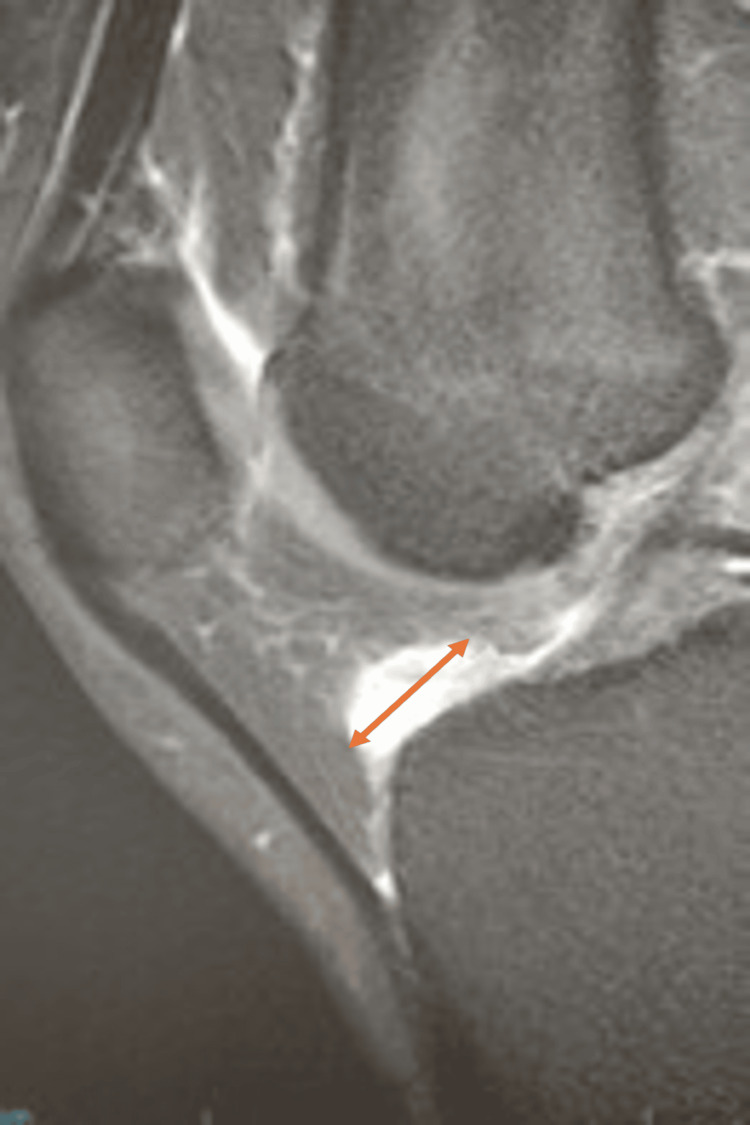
Sagittal T2-weighted MRI showing a well-circumscribed hyperintense ganglion cyst in Hoffa’s fat pad (arrow).
